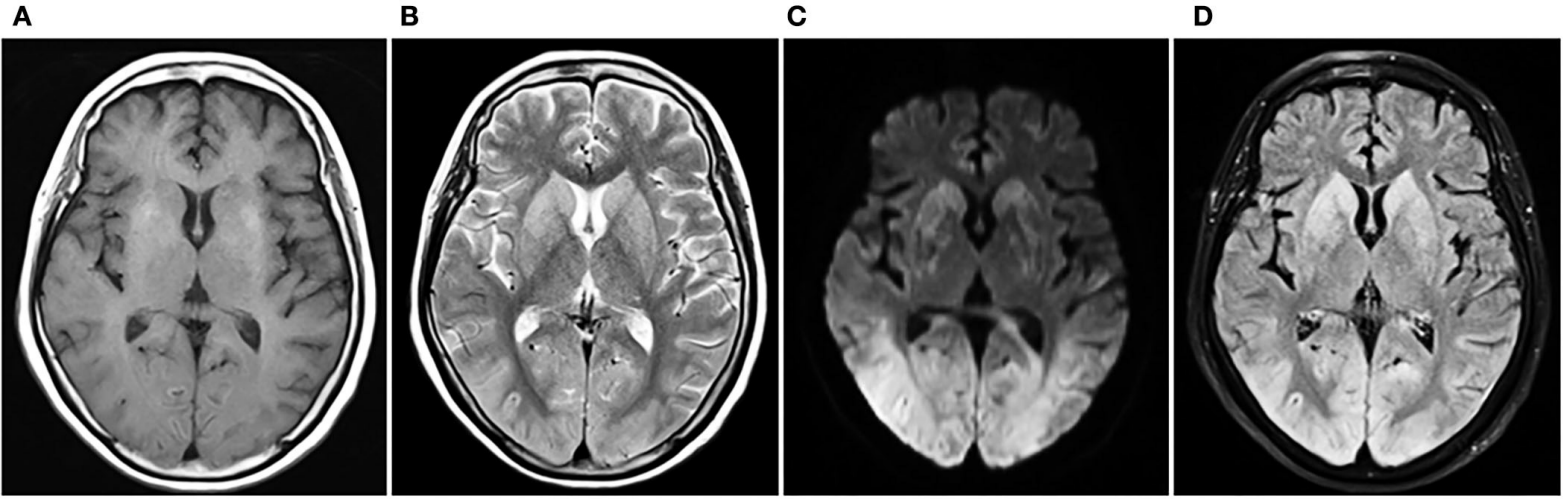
Cerebral MRI contrast of ischemic hypoxic encephalopathy. T2WI and T2-FLAIR show extensive gyral swelling and hyperintensity in basal ganglia bilaterally. DWI demonstrates bilateral symmetrical diffusion restriction throughout the cortex of both cerebral hemispheres, particularly involving the perirolandic and occipital cortices. (A) T1WI, (B) T2WI, (C) DWI, (D) T2-FLAIR. MRI, magnetic resonance imaging; T1WI, T1-weighted imaging; T2WI, T2-weighted imaging; DWI, diffusion-weighted imaging; FLAIR, fluid-attenuated inversion recovery.
